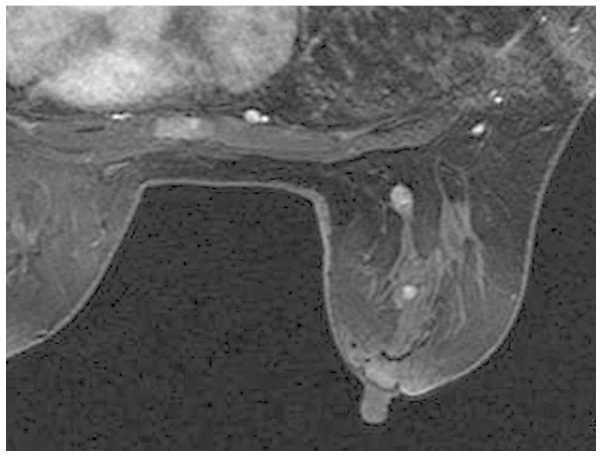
Magnetic resonance imaging revealed two nodules proximal to the areola of the right breast.
